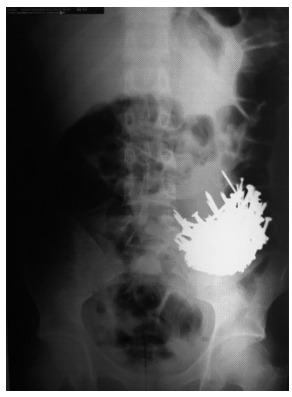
Abdominal radiograph showing strange body image in the left iliac fossa
